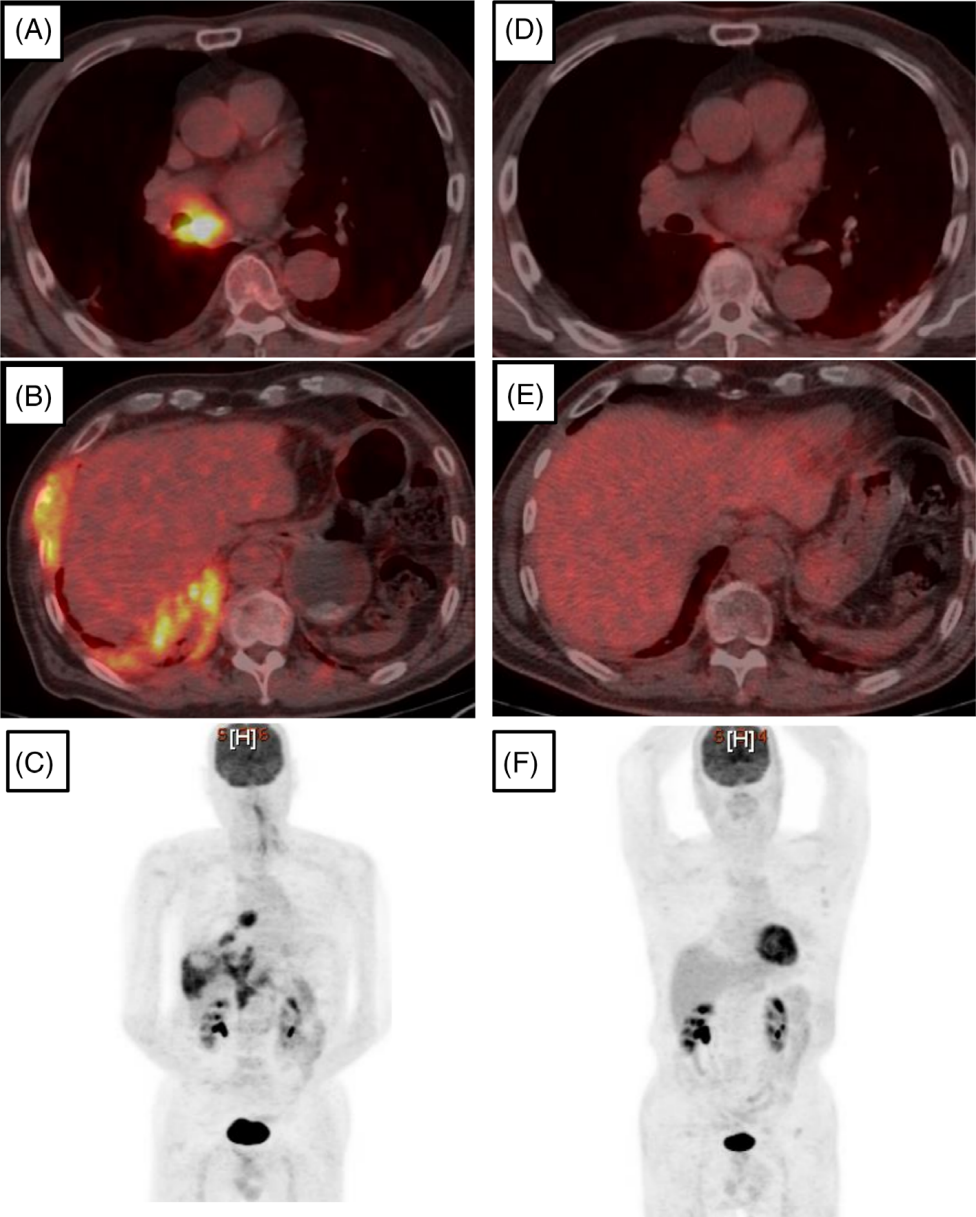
Radiological appearance of the PET scans at the time of the referral and 7 months after the treatment. (A–C) PET scan at the time of referral, showing high uptake of FDG in the mediastinal lymph nodes (SUV max = 11.1) and right pleural thickening (SUV max = 9.2); (D–F) PET scan 7 months after the treatment, showing no abnormal uptake of FDG. FDG, fluorodeoxyglucose; SUV, standardized uptake value; PET, positron emission tomography
